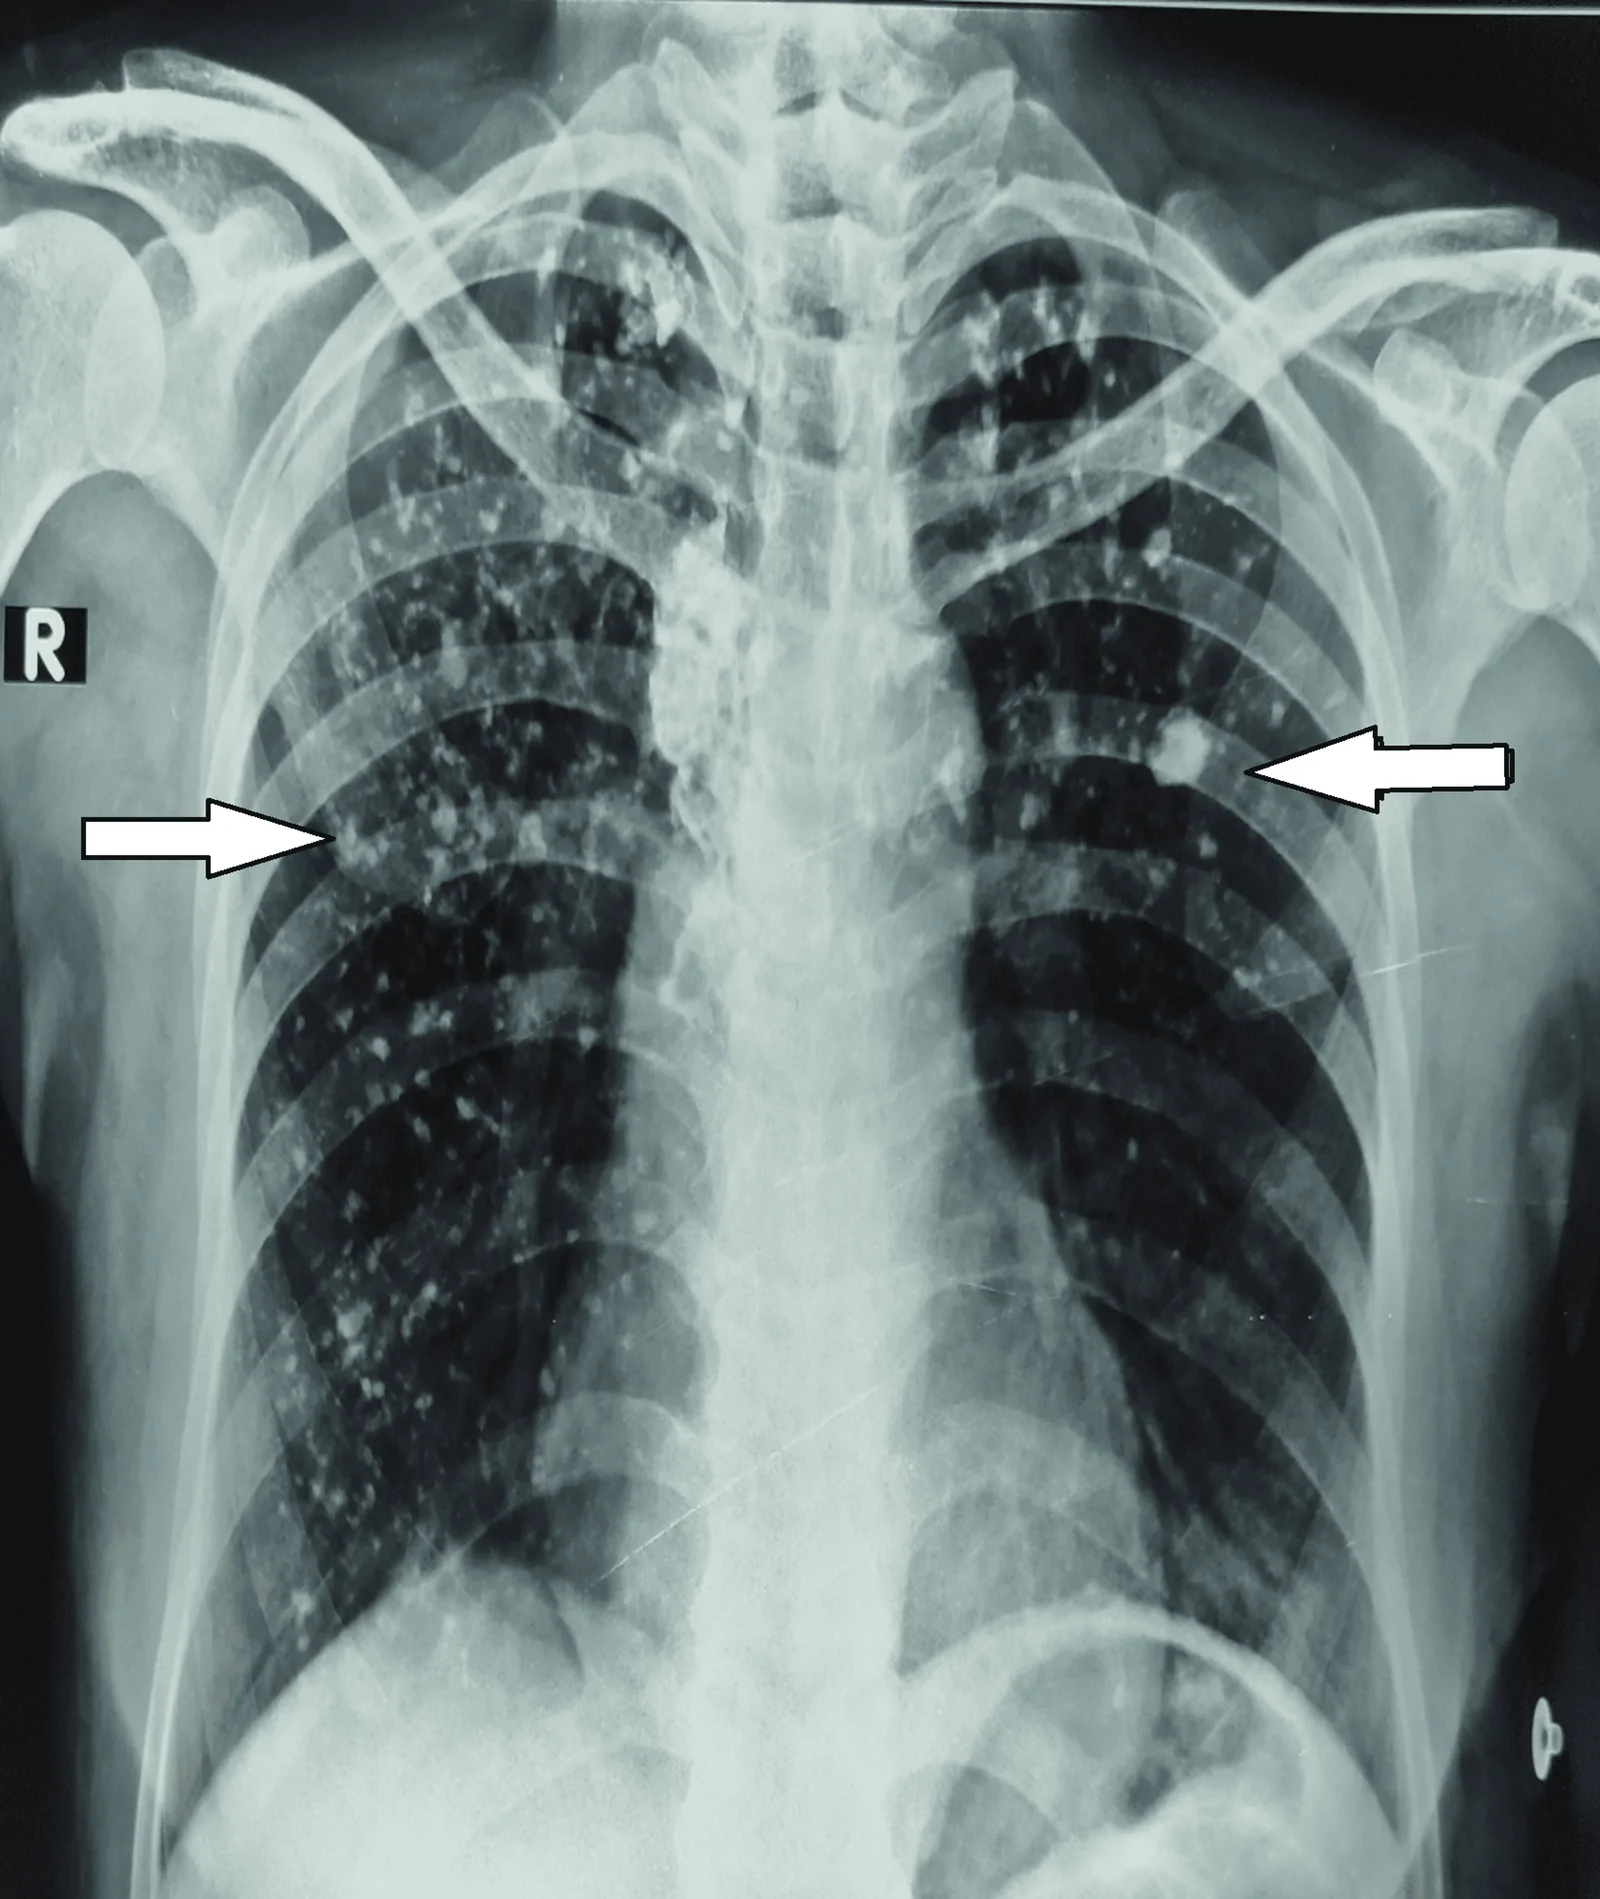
Chest X-ray showing multiple calcified pulmonary nodules Solid white arrows show the multiple healed calcified nodules suggestive of prior pulmonary tuberculosis
